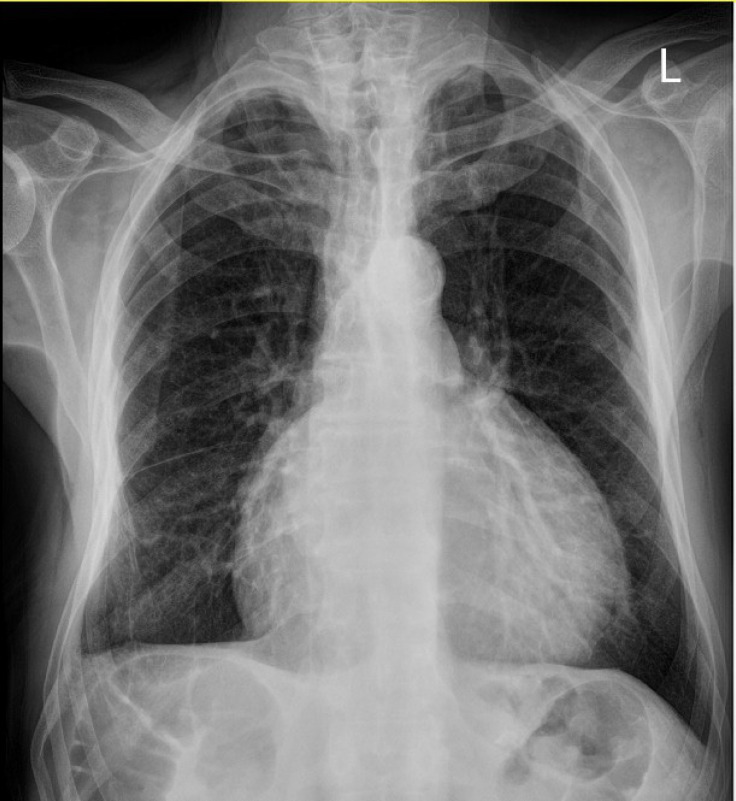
Cardiomegaly in chest X-ray (posterior-anterior view)
